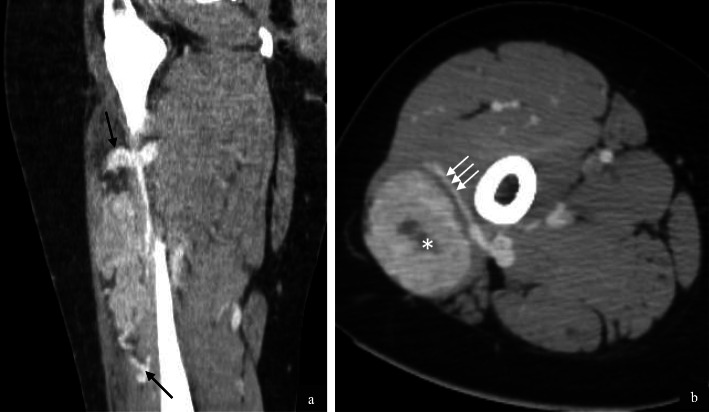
Intramuscular lower limb primary ASPS in a 16 year old female patient. Post contrast coronal (a) and axial (b) CT images demonstrate hypervascularity of the primary tumour. Feeding vessels at the upper and lower poles (a, black arrows) as well as circumferential vessels (b, white arrows) are present. Small volume necrosis is seen centrally (b, white asterisk)
